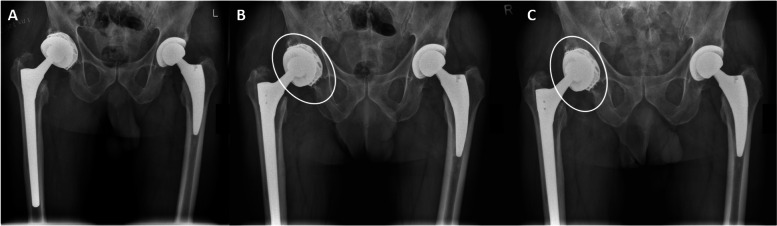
Postoperative radiographs (05/2018) (A), at 3 months postoperatively (B) and at the final follow-up (FU; 04/2020) (C) after “cup-in-cup” revision with a dual mobility cup (DMC). The cup position had already changed at the 3 month-FU (B) and had not further changed at the last FU (C), being at a much steeper position inside the well-fixed cup (white ellipses). This change of position was indicative of loosening of the DMC
